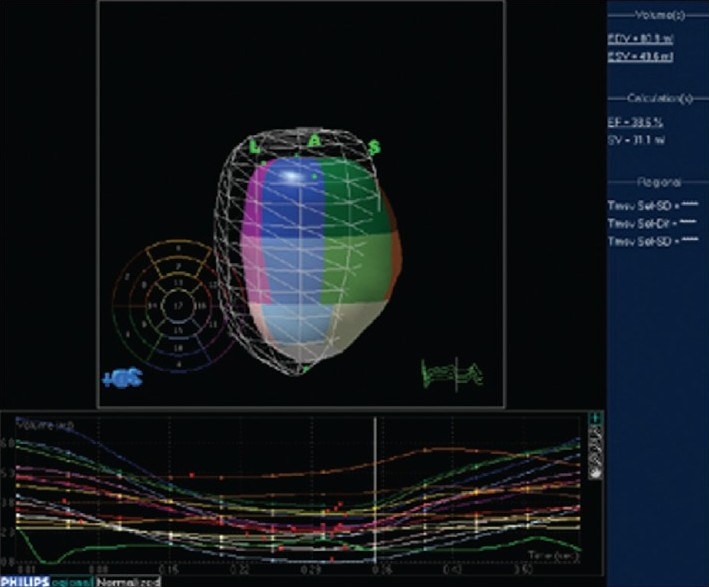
Software for processing 3DE enables quantitation of left ventricular volumes throughout the cardiac cycle, providing enddiastolic and end-systolic volume as well as ejection fraction. The mesh represents left ventricular volume at end-diastole. The cast of the left ventricular cavity consists of segments of varying colors, each of which represents a sub-volume of the ventricular cavity based on the American Society of Echocardiography 16-segment model. The change in volume of each sub-volume is represented graphically, with time on the X axis and volume on the Y axis
